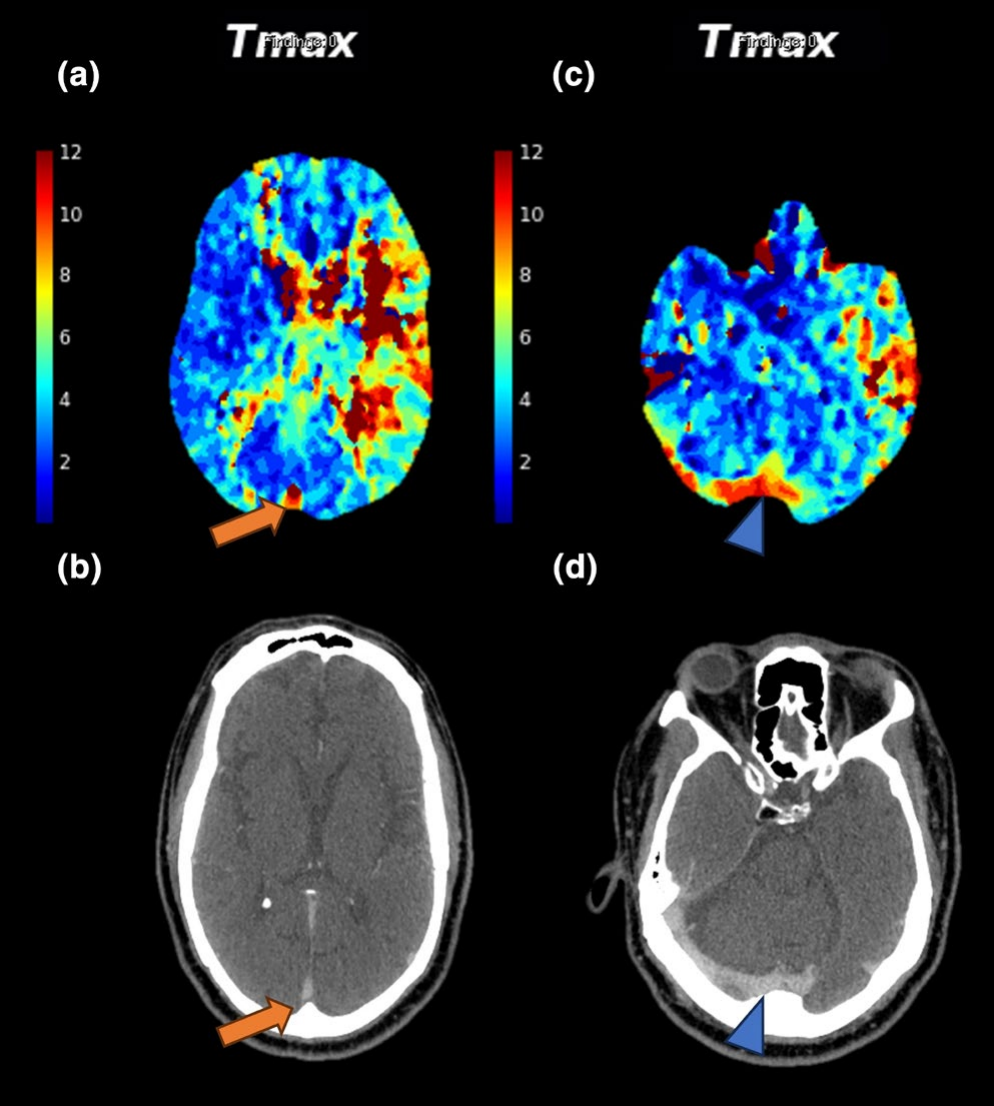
Imaging from a 68‐year‐old man presenting with left M1 occlusion and aphasia. His admission National Institutes of Health Stroke Scale score was 24. Post‐thrombectomy achieved modified Thrombolysis in Cerebral Infarction score 2b reperfusion after one pass. The 90‐day modified Rankin Scale score was 3. Top row: Tmax perfusion maps demonstrating prolonged venous transit in (a) the posterior superior sagittal sinus (orange arrow) and (c) the torcula (blue arrowhead). Bottom row: (b) and (d) corresponding computed tomography angiography images highlighting the same venous structures (arrows).
